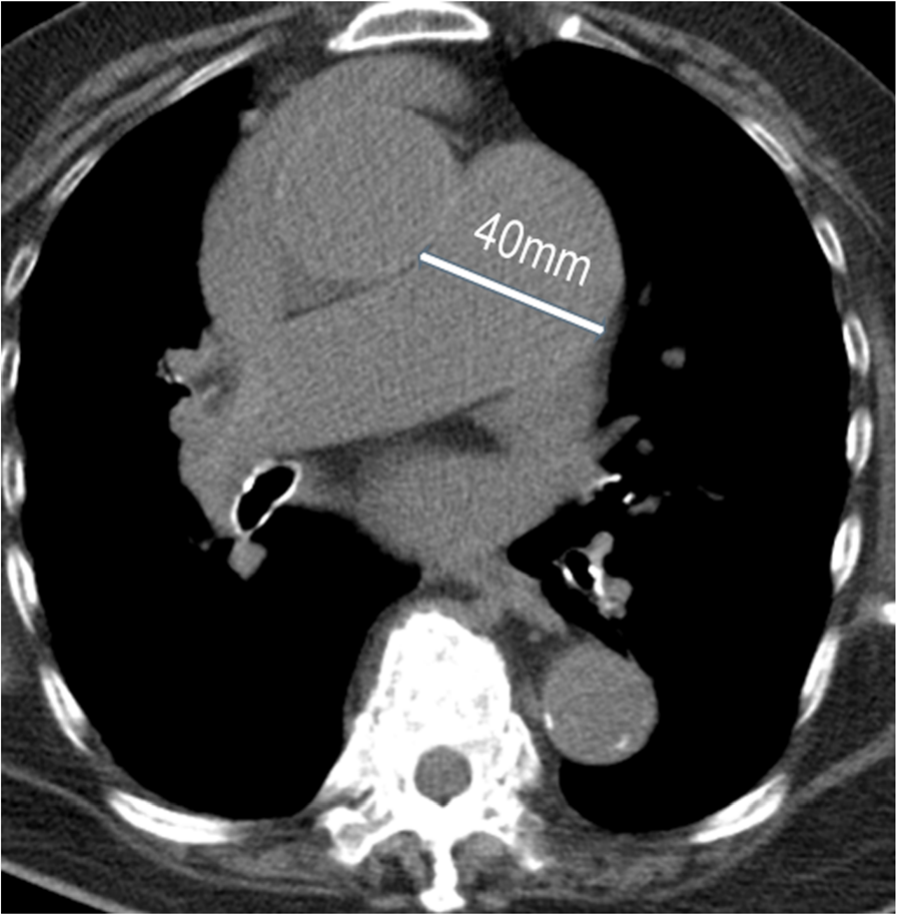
This patient was seen in the emergency department for dyspnea. Computed tomography showed a dilated pulmonary artery (diameter > 33.2 mm)
